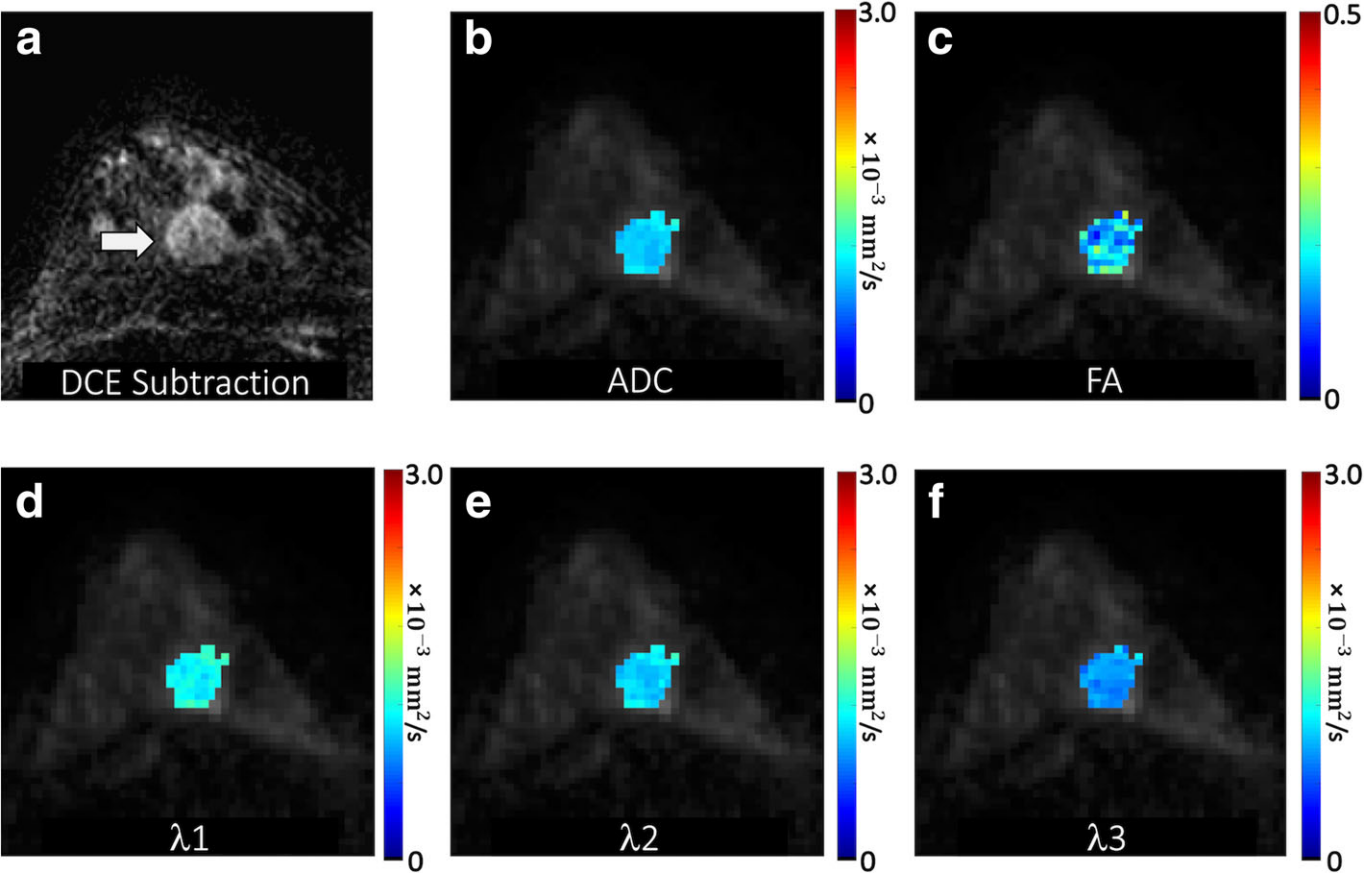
Malignant mass detected in a 47-year-old patient undergoing MRI to evaluate newly diagnosed cancer. a DCE post-contrast subtraction image demonstrates an additional 20-mm round mass with irregular margins in the posterior right breast 6 o’clock (arrow), assigned a BI-RADS category 4. DTI-derived parametric maps of b apparent diffusion coefficient (ADC), c fractional anisotropy, and eigenvalues d
